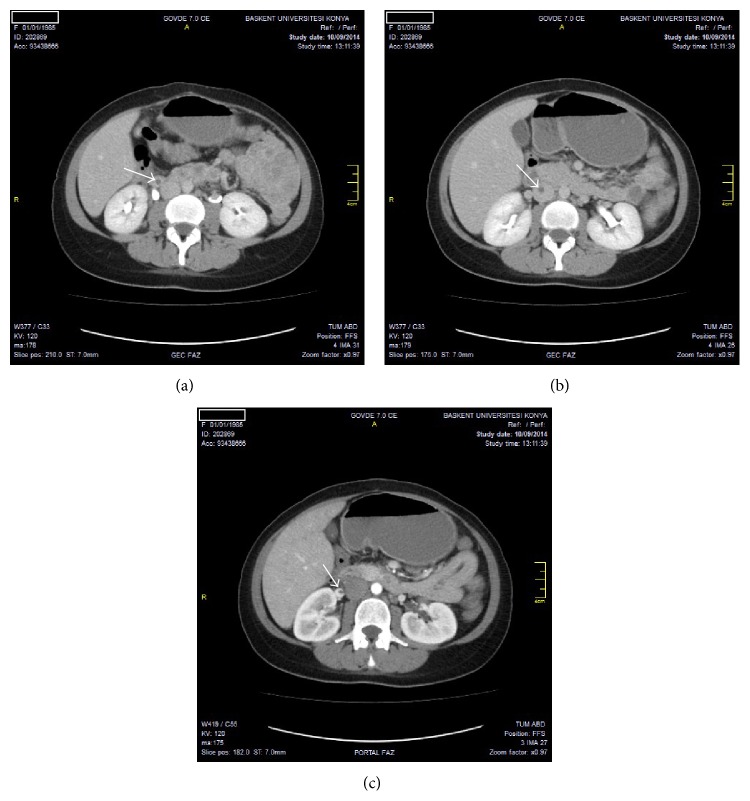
(a) Ovarian vein thromboses. On axial CT image, hypodense thrombus seen in right ovarian vein. (b) Inferior vena cava vein thromboses. On axial CT image, hypodense thrombus seen in vena cava inferior. (c) Renal vein thrombosis. On axial CT image, hypodense thrombus seen in right renal vein.
